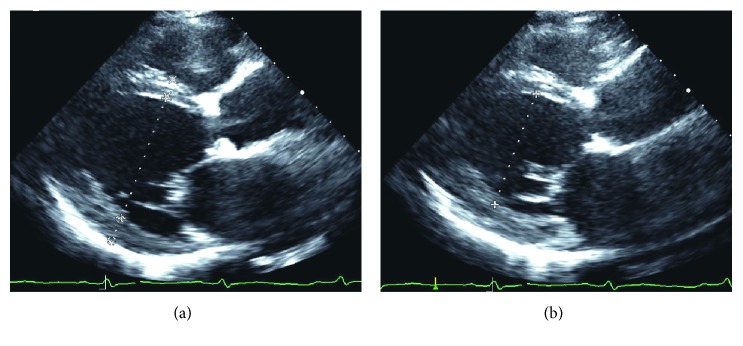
Transthoracic echocardiogram for cardiac size and function. (a) Parasternal long-axis view demonstrating severe dilatation (end-diastolic diameter of 64 mm) with normal anterior septal and inferior lateral wall thickness (9 and 12 mm, respectively). (b) Similar view demonstrating increased end-systolic dimension (57 mm) consistent with reduced ejection fraction.
